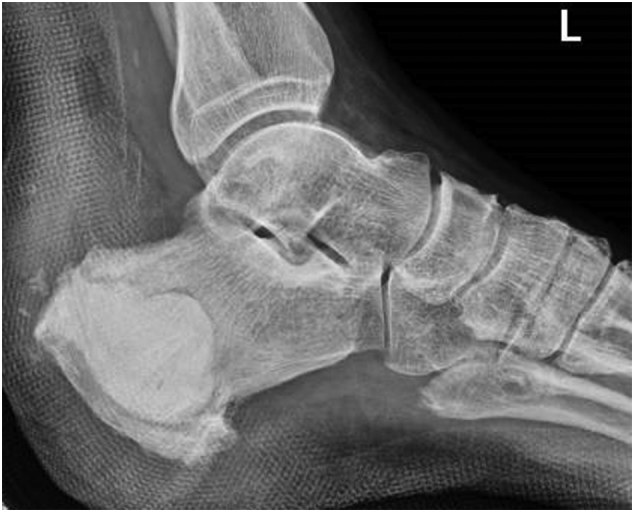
Post-operative radiographs with cement filling the cavity.
